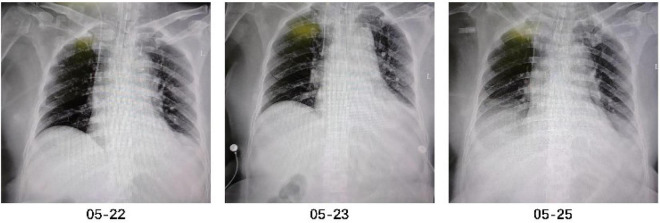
Bedside chest radiographs during hospitalization. The bedside chest radiograph on May 22 showed a small amount of effusion in both lungs and a small amount of pleural effusion on both sides; the radiograph on May 23 showed a small amount of effusion in the left lower lung, a small amount of pleural effusion on the left side, and widening of the right upper mediastinal shadow, which was roughly similar to the radiograph of May 22; the radiograph on May 25 showed a small amount of effusion in both lower lungs and a small amount of pleural effusion on both sides, which was progressive compared to the radiograph of May 23, and widening of the right upper mediastinal shadow, which was similar to the previous radiographs.
